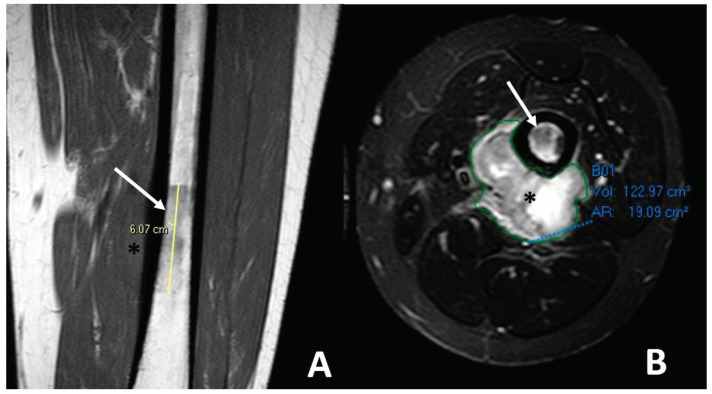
Soft tissue spreading in conventional high-grade osteosarcoma affecting the left femoral bone diaphysis in a 20-year-old male. (A) Coronal T1-weighted imaging (WI) showing the irregular intra-medullary involvement with a low signal intensity (white arrow) and a soft-tissue extension (black asterisk). (B) Axial T2-WI with fat suppression showing ill-defined soft-tissue spreading with high, heterogeneous signal intensities on T2-WI. Of note, the extra-osseous was automatically segmented (green line) for a further volumetric follow-up during neoadjuvant treatments.
